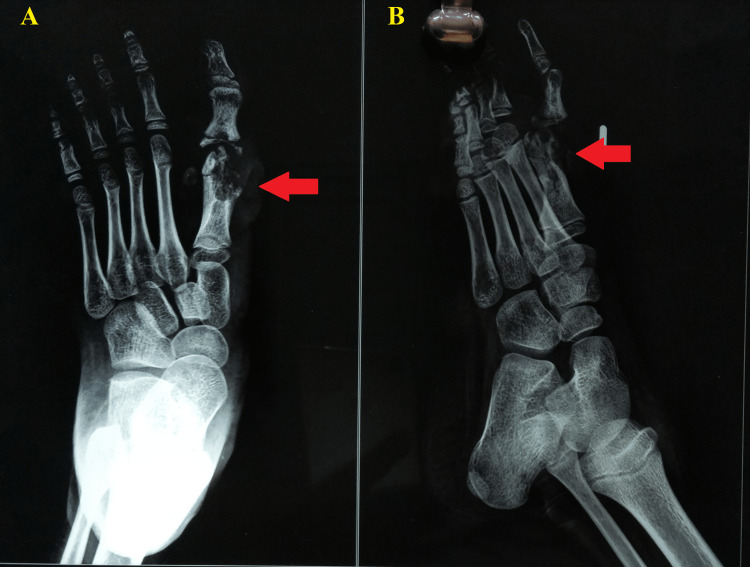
Anteroposterior (A) and oblique (B) radiograph of the left foot.
